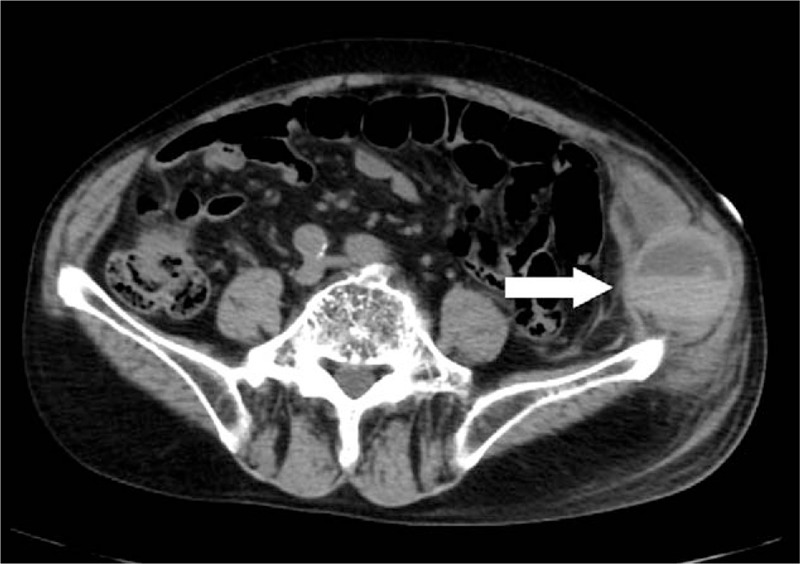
Computed tomography of the abdomen revealed left firmness and intramuscular hematoma (arrow head). Extravasation was not observed using a contrast agent.
